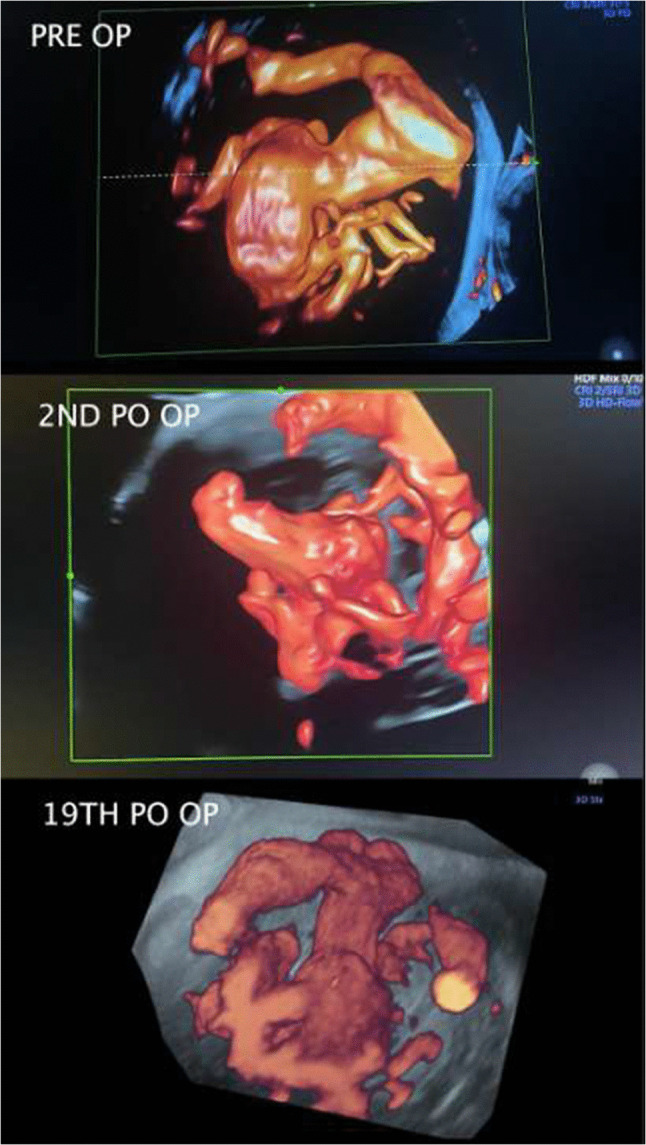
Fetal ultrasound performed on the first, second, and tenth postoperative days following in utero embolization
